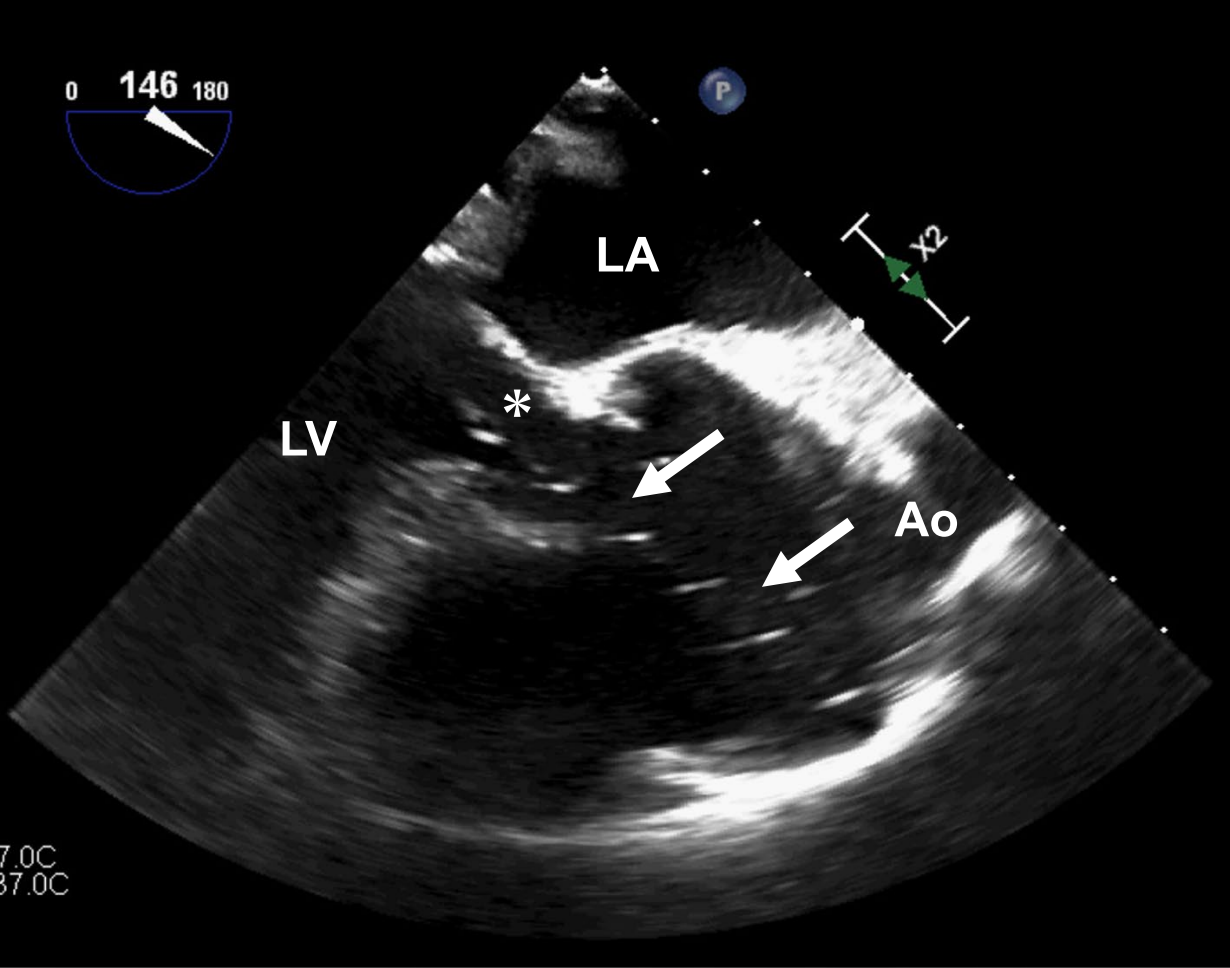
Echocardiographic mid-esophageal aortic valve long-axis view. The tip of the left ventricular vent (white arrow) was detected in the aortic root across the aortic valve (asterisk). Ao, ascending aorta; LA, left atrium; LV, left ventricle
